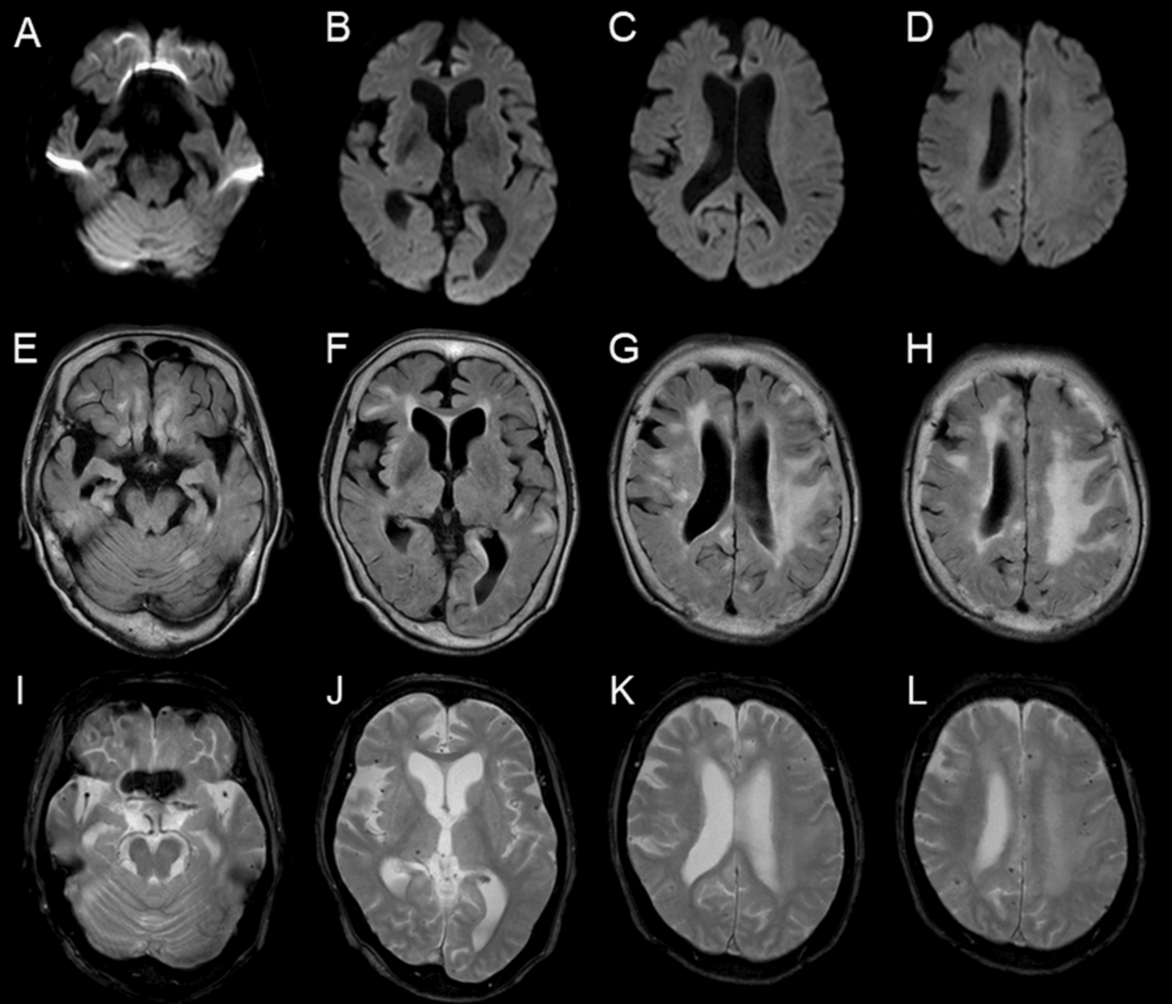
Initial head MRI (A–D) Diffusion-weighted imaging (DWI); (E–H) Fluid-attenuated inversion recovery (FLAIR); (I–L) T2*-weighted imaging DWI showed no abnormalities. FLAIR images demonstrated multiple hyperintense lesions in the cerebellum and bilateral cortical regions. T2*-weighted images revealed multiple cerebral microbleeds in the right thalamus and bilateral cortical and subcortical areas.
